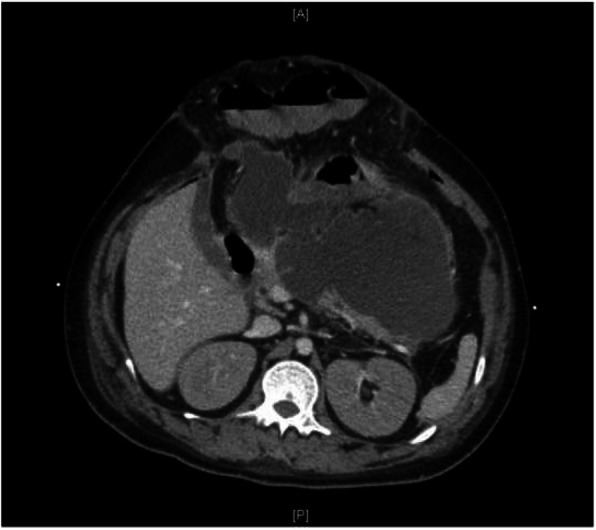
Computed tomography of the abdomen a month after surgical decompression revealed a large retroperitoneal collection seen within lesser sac between pancreatic tail and stomach, consistent with walled-off necrosis. Axial view
